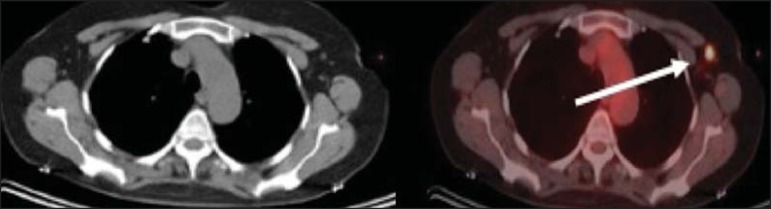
A 57-year-old woman with breast cancer and focal FDG uptake in a left
